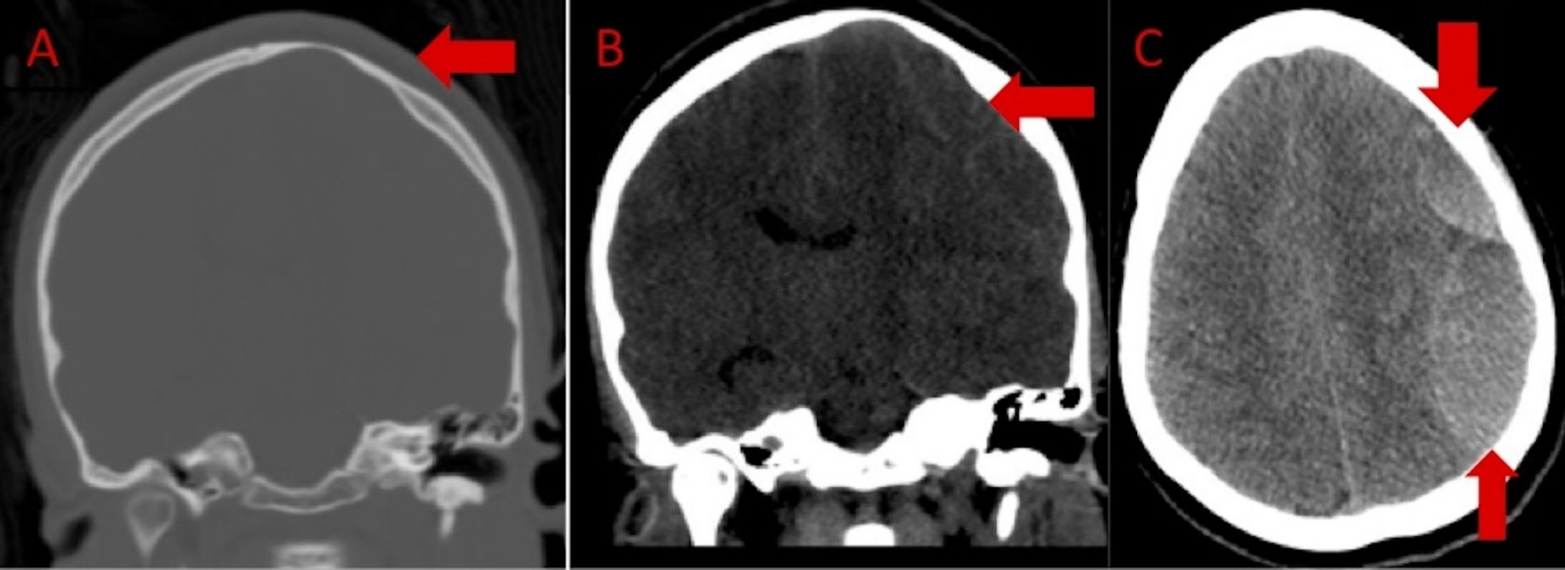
Admission computed tomography of the head A: Coronal view bone window sequence demonstrating scalloping of the calvarium (arrow) in the region of the known left frontal arachnoid cyst. B: Coronal view demonstrating an isodense left frontal extra-axial fluid collection {arrow) with mass effect upon the left cerebral hemisphere with resultant left-to-right midline shift. C: Axial view demonstrating a loculated hyperdense (up-arrow) and isodense extra-axial fluid collection in the region of prior AC, suspicious for intra-cystic hemorrhage (down-arrow) and subdural hematoma.
